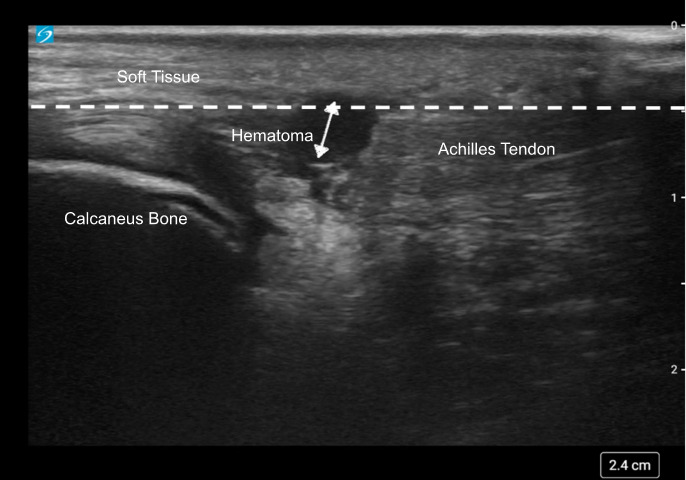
Point of care ultrasound (POCUS) image showing a sagittal view of a lacerated Achilles tendon (tendon below the dotted line) with a hematoma (double arrows above the laceration).
